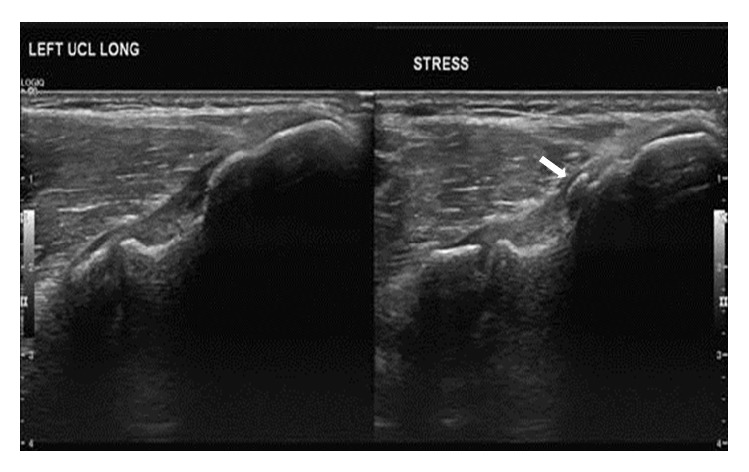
Preoperative ultrasound. Images demonstrate mild thickening of the proximal fibers of the proximal ulnar collateral ligament (medial epicondyle is to the right of image, and ulna is to the left of the image), likely sequela of prior or chronic repetitive low grade injury. Small area of heterotopic ossification embedded within the proximal fibers of the ulnar collateral ligament (arrow). Dynamic stress images demonstrate mild “gapping” of the medial compartment (2 mm) suggestive of laxity.
